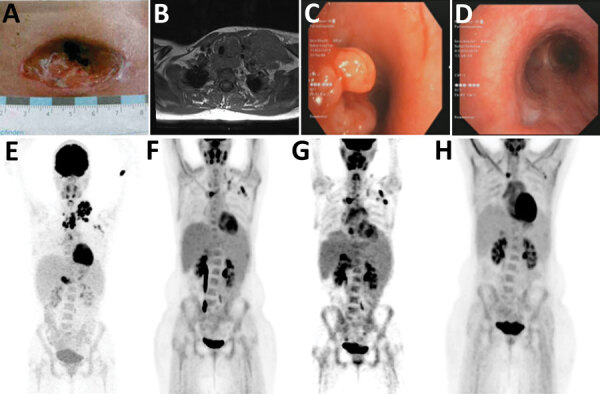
Clinical manifestations and radiologic findings in the course of disease in a 23-year-old woman with disseminated *M. genavense* infection preceding Hodgkin lymphoma, Germany. A) Cervical wound after initial lymph node extirpation. B) Magnetic resonance imaging at the time of initial evaluation. C) Endobronchial view of tracheo-esophageal fistula before positioning of a stent. D) Endobronchial view of the prior tracheo-esophageal fistula after treatment. Whitish scar tissue is seen at the bottom left. E) ^18^F-FDG-PET scan at initial evaluation (maximum intensity projection). Cervical lymph node mass is seen, with no pathologic uptake in the abdomen. F) ^18^F-FDG-PET scan after 6 months of antibiotic treatment showing reduced uptake. G) ^18^F-FDG-PET scan shortly before Hodgkin lymphoma was diagnosed showing new hepatosplenomegaly and lymphadenopathy. H) ^18^F-FDG-PET scan after antibiotic and chemotherapy without pathologic enhancement.
